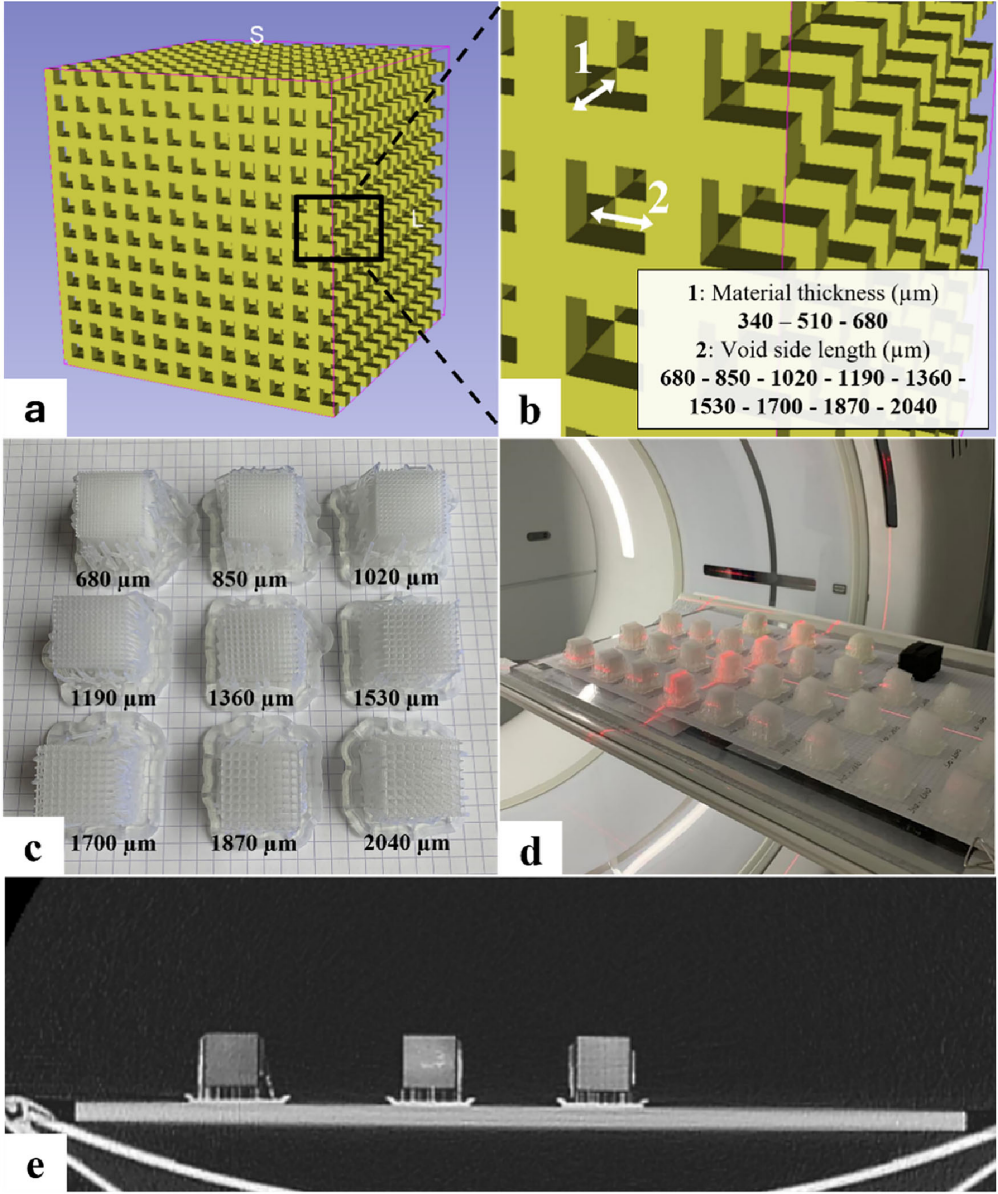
Design and characterisation of cubic SLA-printed samples. a Designed cubic samples with a lattice structure. b Magnified view of the 3D-beams of variable thickness (1: material thickness) placed into a 3D-grid of variable spacing (2: Void side length). c Picture of cubic samples printed with a material thickness of 340 µm and varying gaps. d Image acquisition of the 3D-printed samples on a 256-slice CT scanner (GE Healthcare Revolution). e Example of a CT image slice used for HU characterisation. 3D, Three-dimensional; CT, Computed tomography
